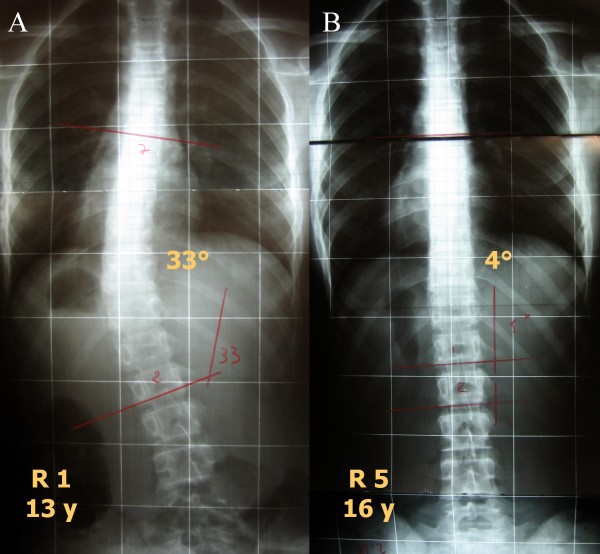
One of the best results obtained in this study. A. Starting x rays. B. Final x-rays. P decreased from 33° at 13 years of age, Risser 1, pre-menarchal to 4° at 16 years, Risser 5.
